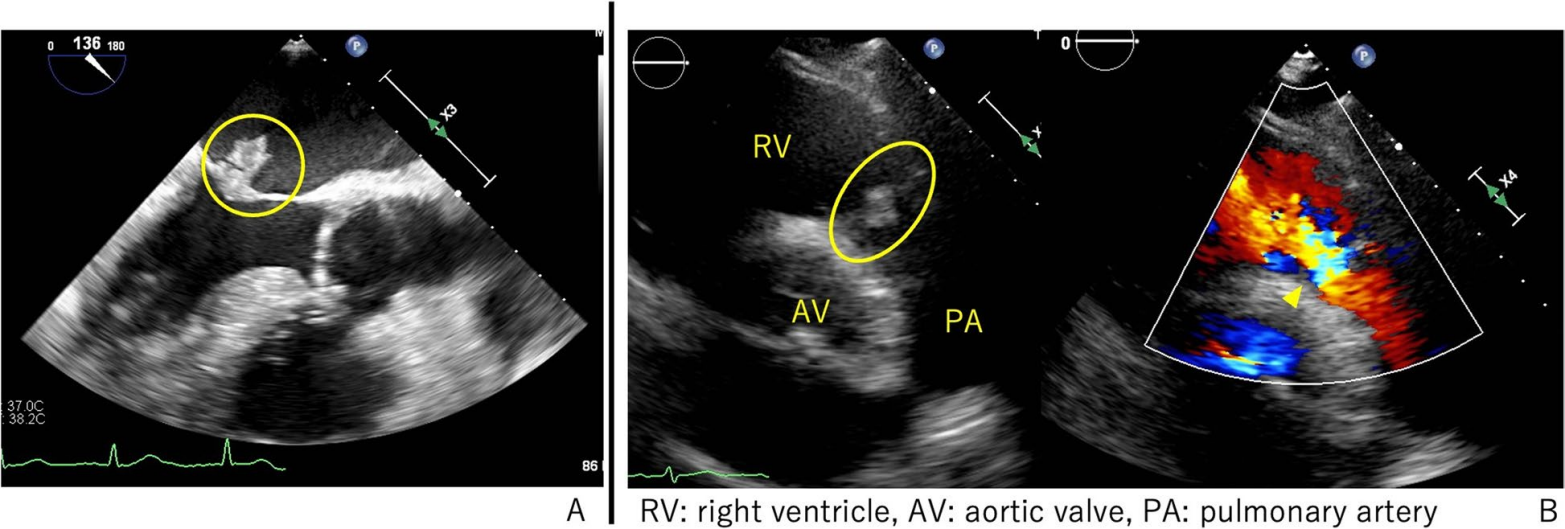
Preoperative echocardiography. A There was 10 mm vegetation adherent to the mitral valve (circle). B There was a thickening of pulmonary valve (oval) and moderate pulmonary valve regurgitation (arrowhead)
